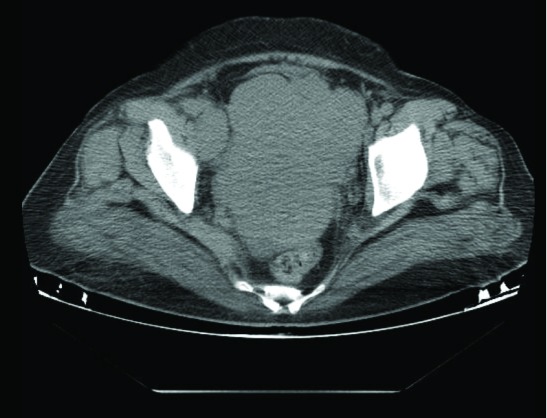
Abdominopelvic CT. A soft tissue mass in the pelvic cavity with right external iliac and para-aortic lymphadenopathy.
